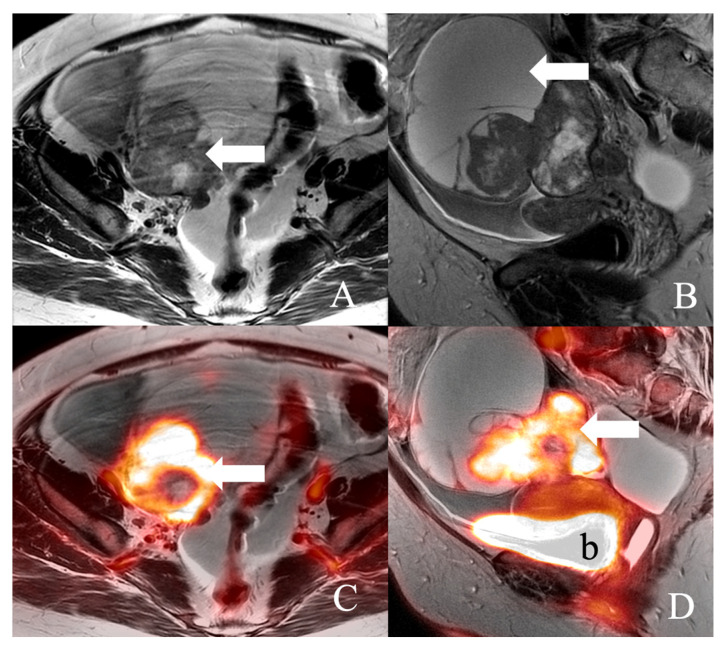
49-year-old female with high-grade Mullerian carcinoma of the right ovary. (A) Axial T2WI, (B) Sagittal T2WI MR images, and (C) Axial fused T2WI. (D) Sagittal fused T2WI positron emission tomography/MRI images demonstrate a large complex right adnexal mass (arrow) with an enhancing soft tissue component within the mass. b, urinary bladder.
